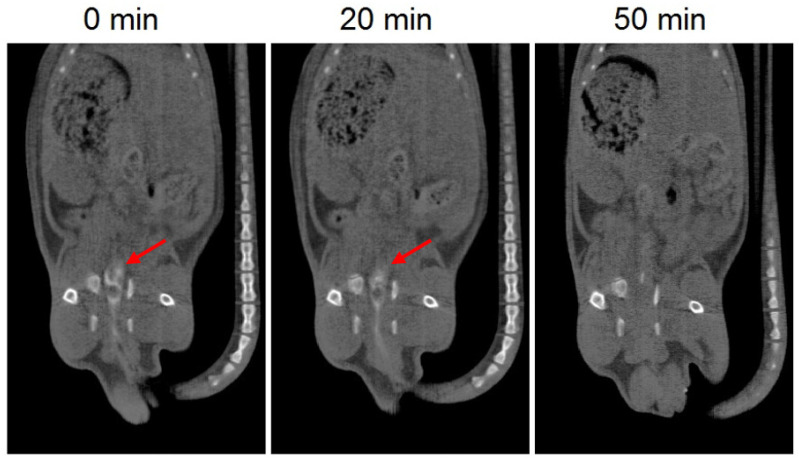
CT images of 3D printed suppositories with barium sulphate at different times. The red arrow indicates the location of the suppository (white color).
